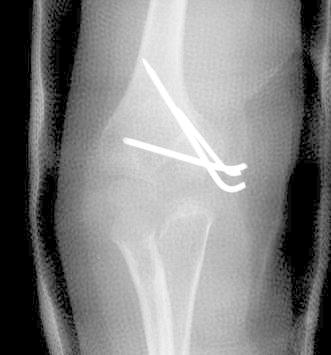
Postoperative anteroposterior radiograph. The cartilaginous trochlea was fixed with three smooth Kirschner wires. A long-arm cast was applied with the elbow fixed at 90° and the forearm in neutral position, and then was removed after 6 weeks
